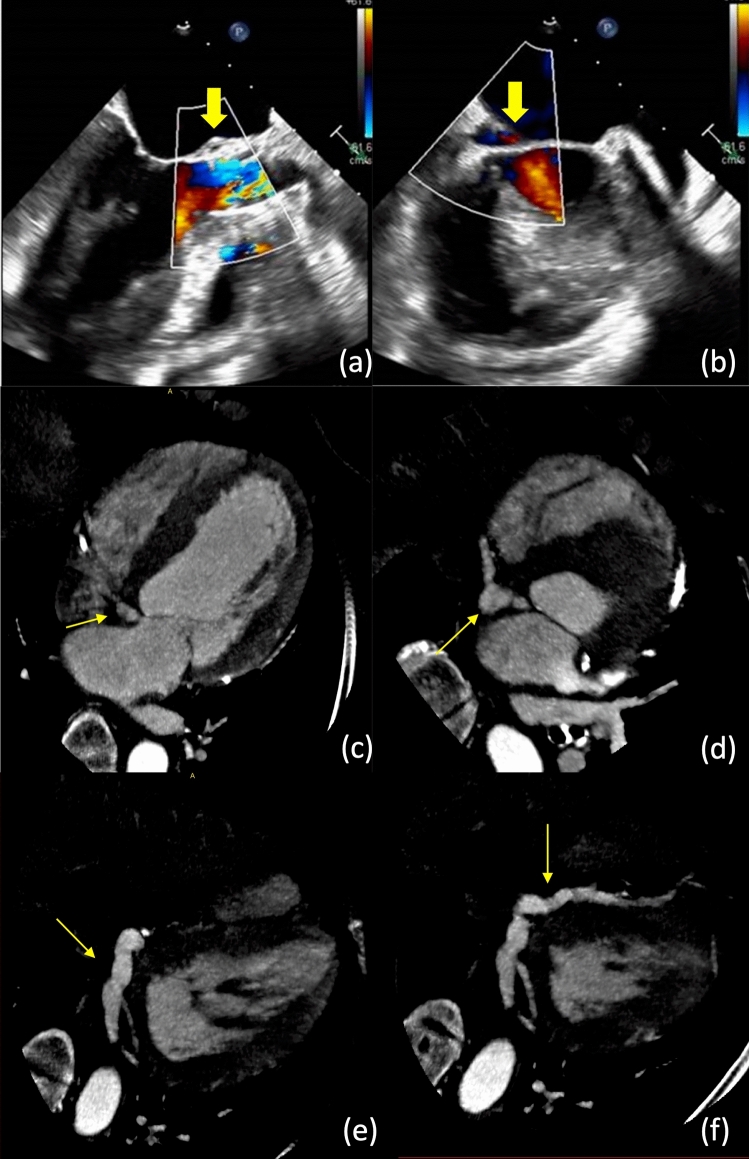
a Left ventricular and aortic valve transesophageal echocardiogram (TEE) view showing area of echolucency (yellow arrow) in the posterior aortic annulus area, bulging in systole (3-4 mm) in the right fibrous trigone. b In the same view as above, the echolucnecy is seen extending laterally and into the atrioventricular septum and lower atrial septum. c−f Serial cardiac computed tomography (CT) imaging (axial view) confirming the pseudoaneurysm (yellow arrow) communicates with the left ventricular outflow tract and travels posteriorly with connection to the left atrium, which then travels inferiorly with a pseudovascular distribution that occupies the space within the inferior interventricular groove
